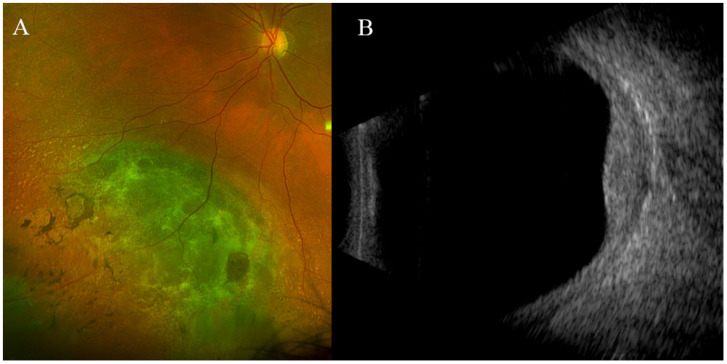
Imaging test results of the choroidal pigment mass under study in patient BUM4. (A) Eye fundus showing a mass with mixed pigmentation. (B) Ocular ultrasound of the LE displaying a choroidal mass compatible with uveal melanoma, measuring 3.6 mm in height and 14.3 mm in base.
